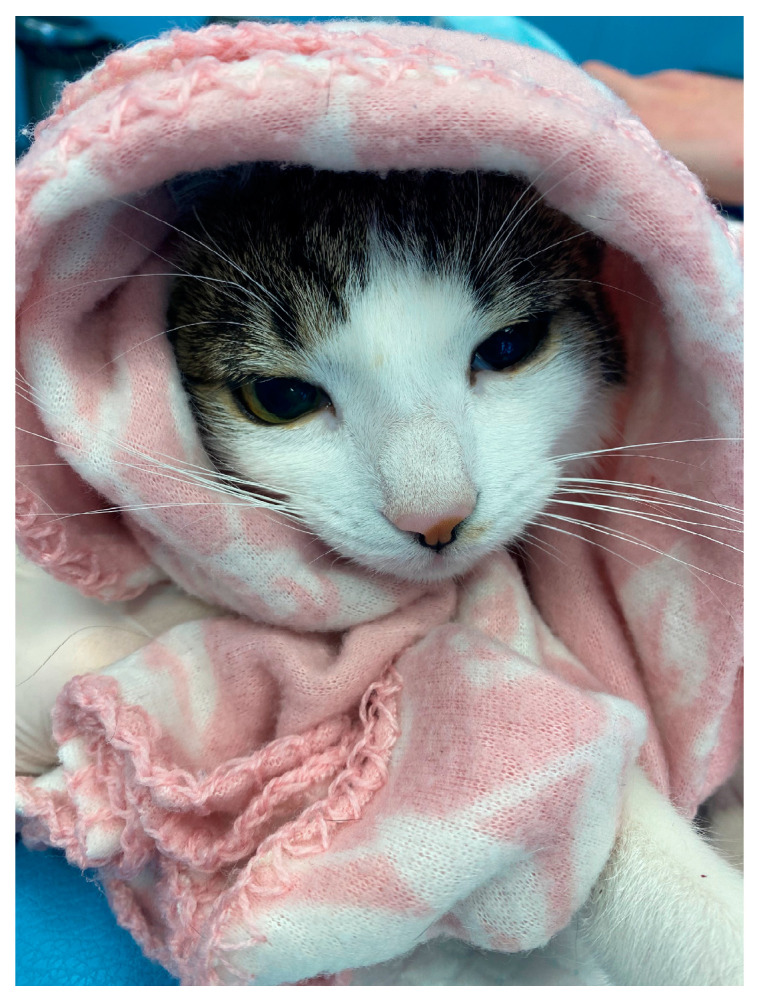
Patient from G1 in the recovery time after the MRI examination.
